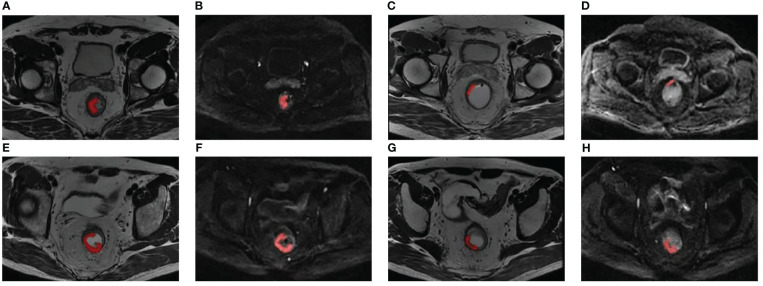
Two examples of segmentation of ROIs on the pre- and posttreatment images. Panels (A-D) show the segmentation of a 51-year-old patient with low-rectum adenocarcinoma at a stage of cT3N1M0. (A, B) Pretreatment oblique axial T2WI and axial DWI (b = 1000 s/mm^2^); (C, D) Posttreatment oblique axial T2WI and axial DWI (b = 1000 s/mm^2^); this patient was demonstrated pCR by surgical pathology. Panels (E-H) show the segmentation of a 60-year-old patient with low-rectum adenocarcinoma at a stage of cT3N1M0. (E, F) Pretreatment oblique axial T2WI and axial DWI (b = 1000 s/mm^2^); (G, H) Posttreatment oblique axial T2WI and axial DWI (b = 1000 s/mm^2^); this patient was demonstrated non-pCR by surgical pathology. DWI, diffusion-weighted imaging; pCR, pathological complete response; ROI, region of interest; T2WI, T2-weighted imaging.
